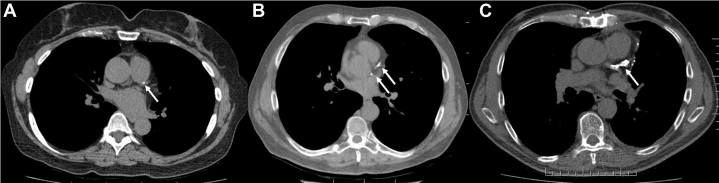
Examples of Incidental Coronary Calcium Identified on Chest CT Scans Examples of mild (A), moderate (B), and severe (C) visual coronary calcium are shown. White arrows point to the location of the coronary calcium, which was in the left anterior descending coronary artery in all 3 patients.
